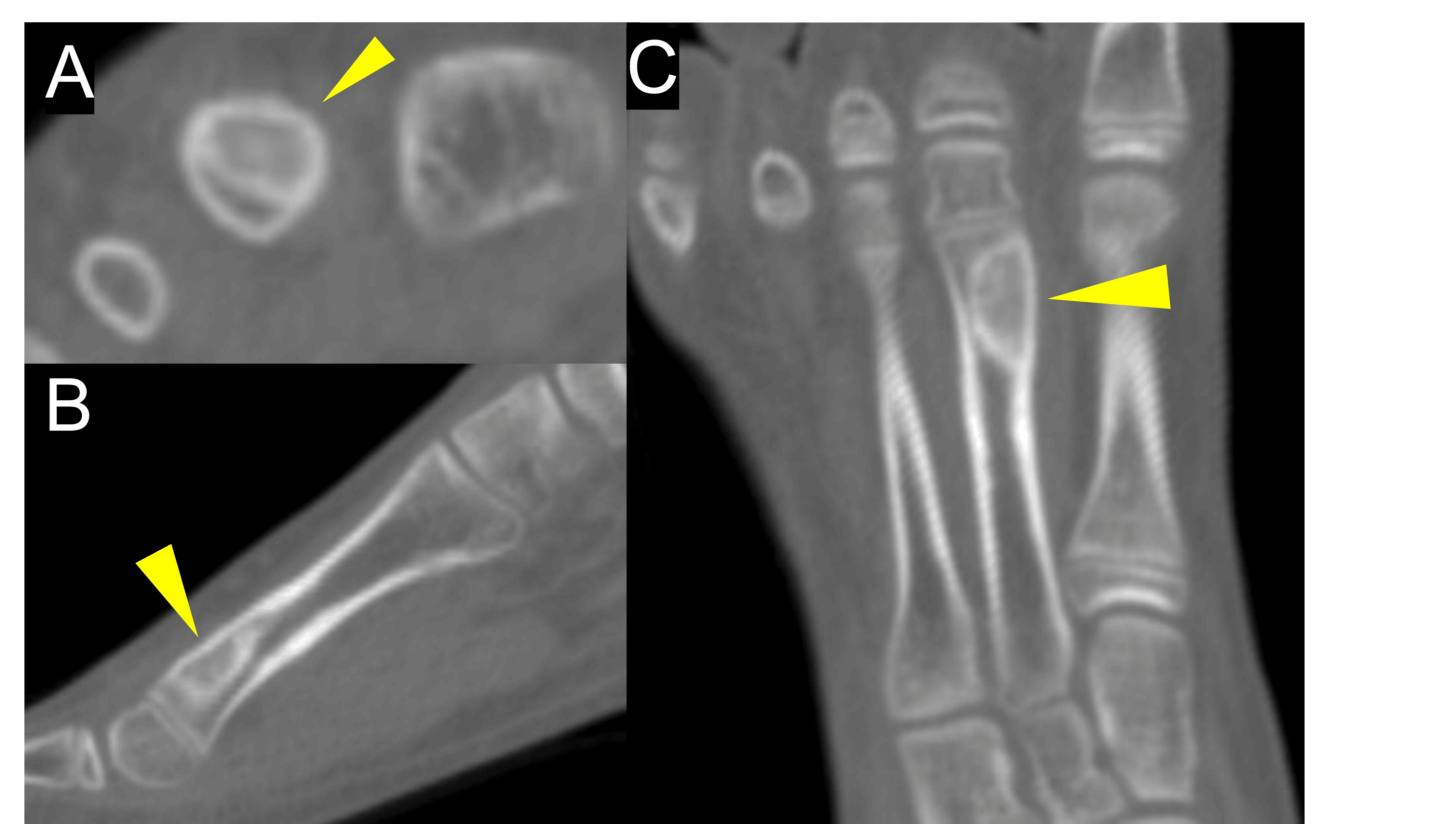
Computed tomography images obtained six months after surgery Computed tomography images obtained six months after surgery in a 12-year-old boy with a giant cell tumor of bone in the right second metatarsal. (A) Axial, (B) sagittal, and (C) coronal views. Arrowheads indicate the OCP/Gel graft site following tumor curettage.
